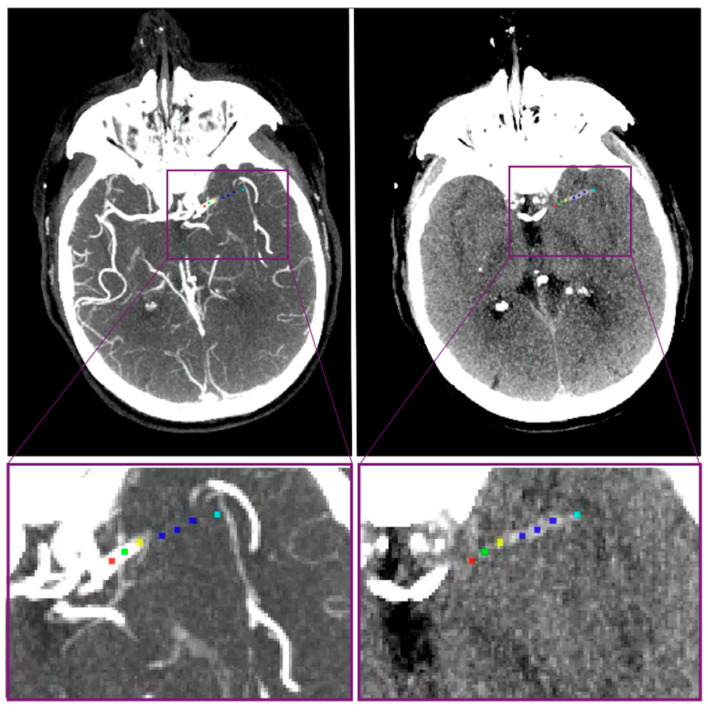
Thrombus imaging characteristics. Manually placed markers to compute thrombus characteristics. Left: thick-slab maximum intensity projection of a CT angiography showing a sudden stop of contrast due to the occlusion. Right: thick-slab maximum intensity projection of a non-contrast CT where the hyperdense artery sign of the thrombus is visible. Red marker: placed at internal carotid artery terminus (ICA-T). Green markers: placed between ICA-T and proximal border of the thrombus. Yellow marker: proximal thrombus border. Blue markers: proximal, mid, and distal parts of the thrombus. Cyan marker: distal thrombus border.
